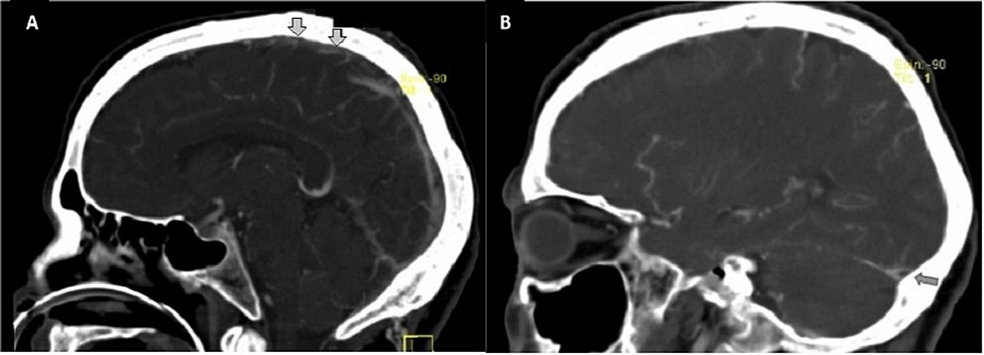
Cerebral veno-CT findings. Cerebral veno-CT confirmed the absence of filling of the superior longitudinal sinus (A) and the right lateral sinus (B) CT: computed tomography
